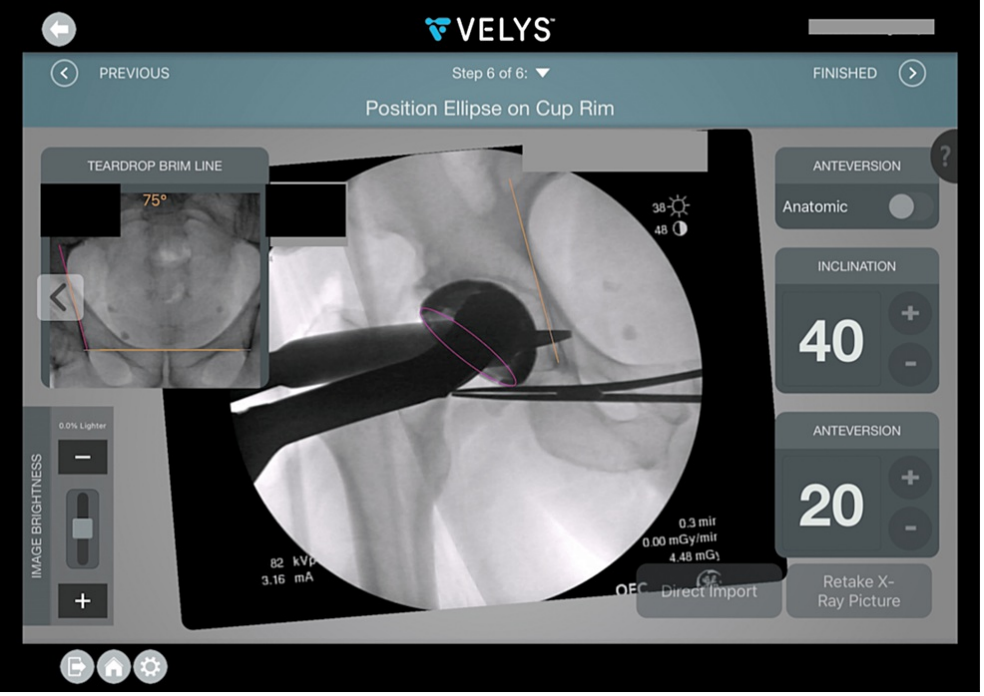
Screenshot of Velys™ intraoperative with acetabular cup trial based on pelvic landmarks determined from initial pelvis radiograph.
